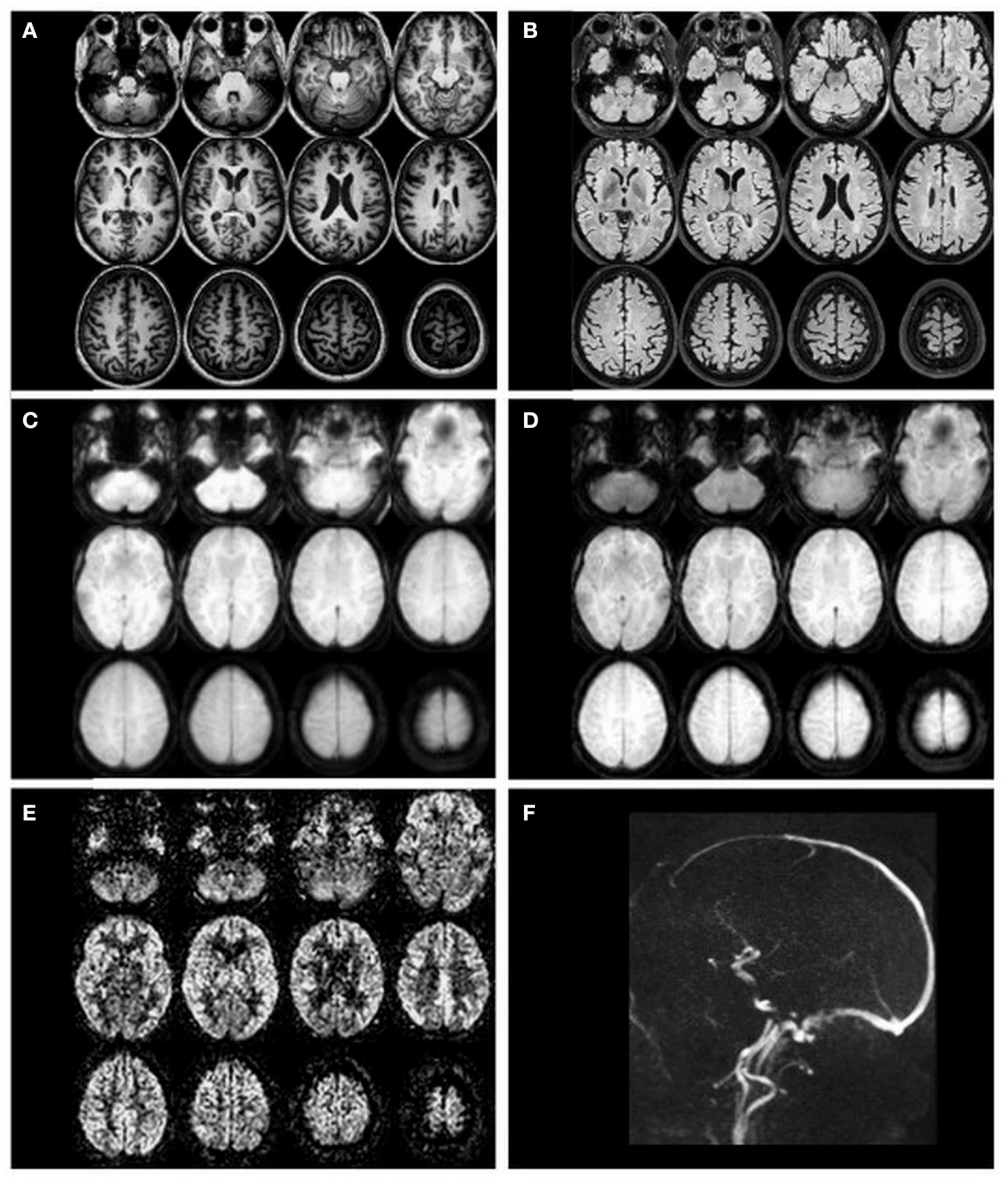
Example transverse slices of intermediate and final ASL images as quality control provided by ExploreASL, from a single healthy volunteer (female, 79 years of age) with 2D EPI PCASL sequence. (A) T1-weighted structural image in standard space; (B) T2 FLAIR image (pre-processed); (C) M0 image; (D) an average raw non-subtracted ASL control image; (E) the final CBF image; (F) a sagittal 2D time-of-flight vessel scout used to position the labeling plane.
